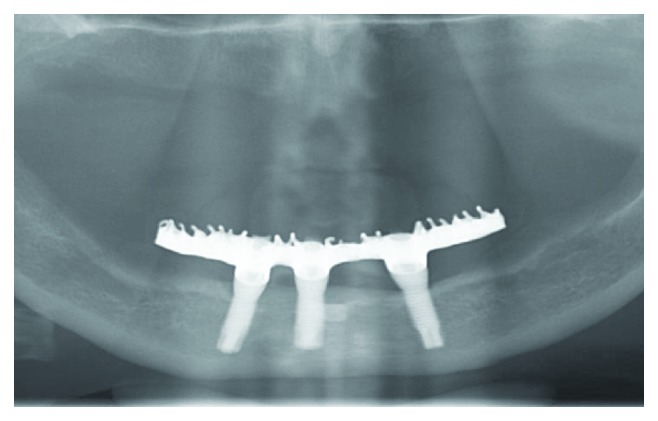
Eight-year follow-up panoramic radiograph: note that there is no bone loss around the implants.
